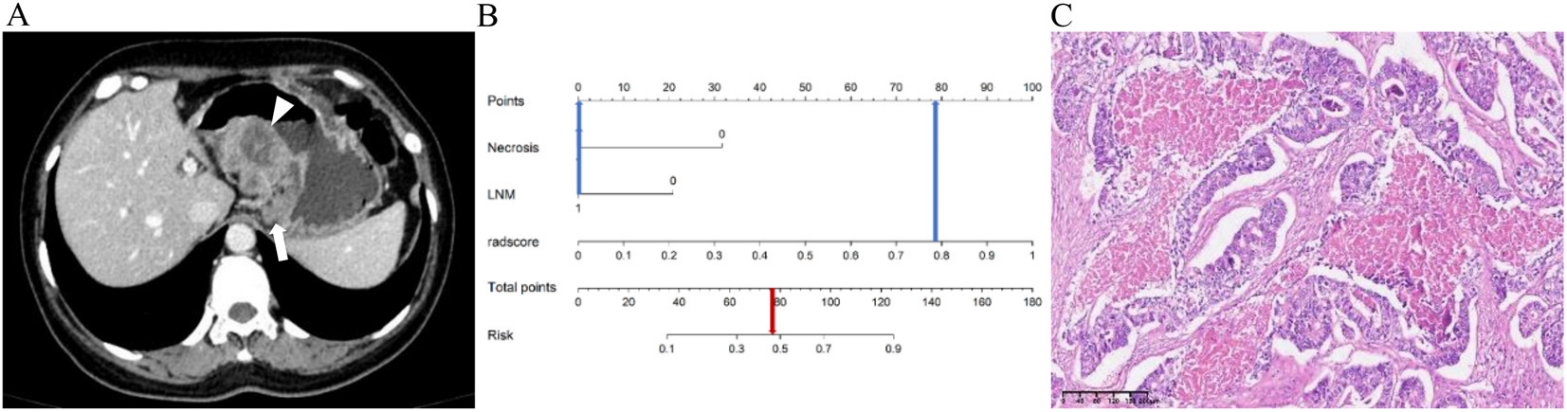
A 48-year-old female patient with g-ADC. (A) Axial CT image in the portal venous phase showed the thickening of the gastric wall in the middle part of the stomach with necrosis (arrowheads), and enlarged lymph node along the lesser curvature of the stomach (arrow) with a short diameter of 1.7cm. (B) The predicted value of Risk in the Nomogram exceeds the critical point (0.36), therefore the diagnosis is determined as g-ADC. (C) The pathological diagnosis is confirmed as g-ADC (HE, ×100).
